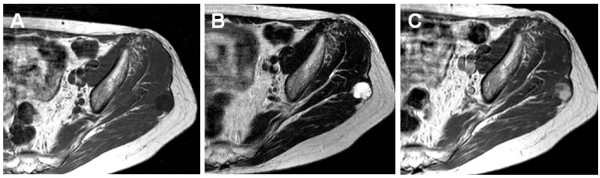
Magnetic resonance imaging demonstrates a well-defined soft tissue mass in the left gluteus maximus. The mass shows homogenous low signal intensity on T1-weighted images (A) and markedly high signal intensity on T2-weighted images (B). Axial T1-weighted images after gadolinium administration reveal heterogenous enhancement throughout the mass (C).
